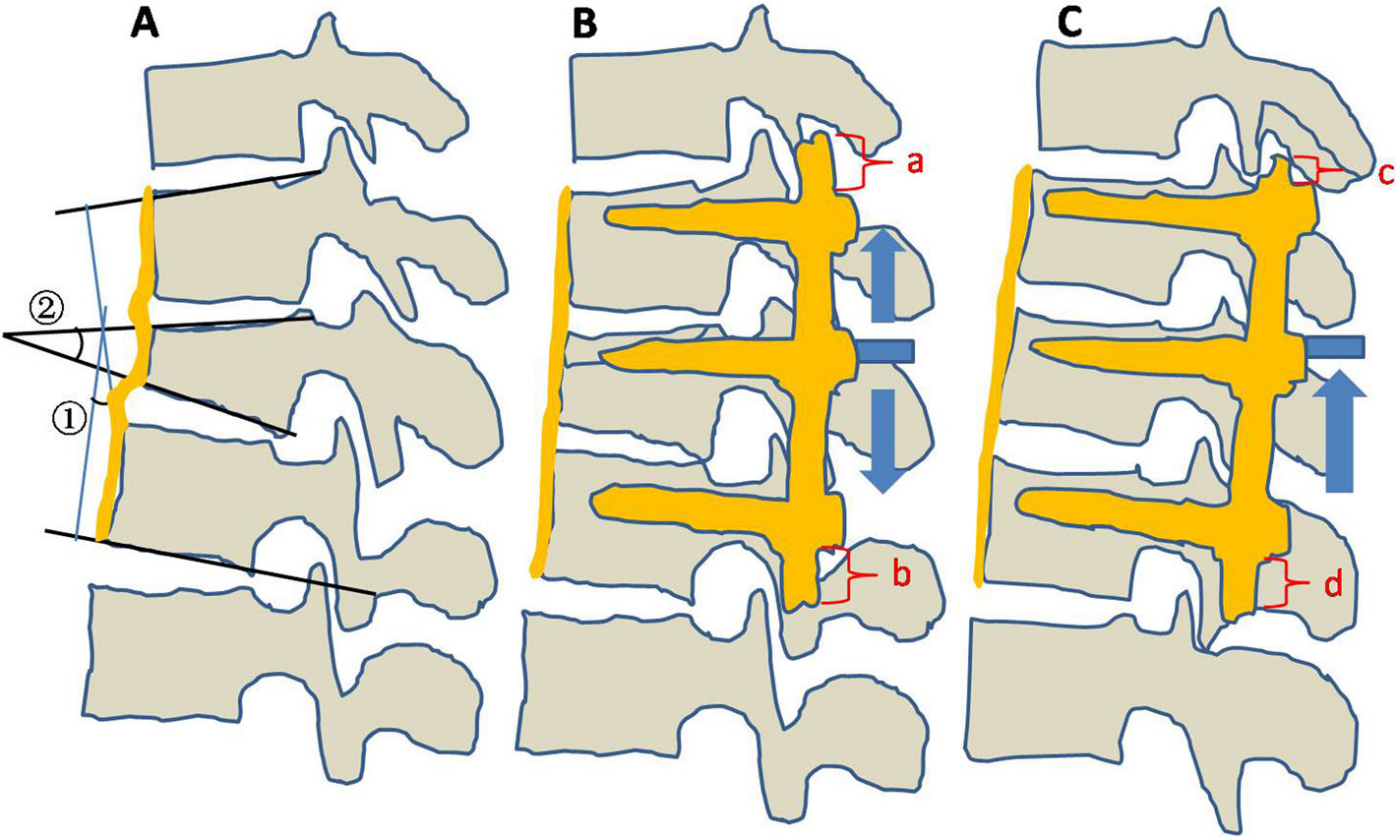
a. Radiological evaluation using plain lateral radiography. ①:Kyphosis angle, ②:vertebral wedge angle; The anterior longitudinal ligament is curly in fracture region. b. Correction of the segmental kyphotic angle was performed indirectly via connecting rods distraction proximally and distally in sequence. The ALL and PLL were straightened as the ligamentotaxis. c. Reduction of the endplate collapse was performed directly by longitudinal compression of intermediate screws
